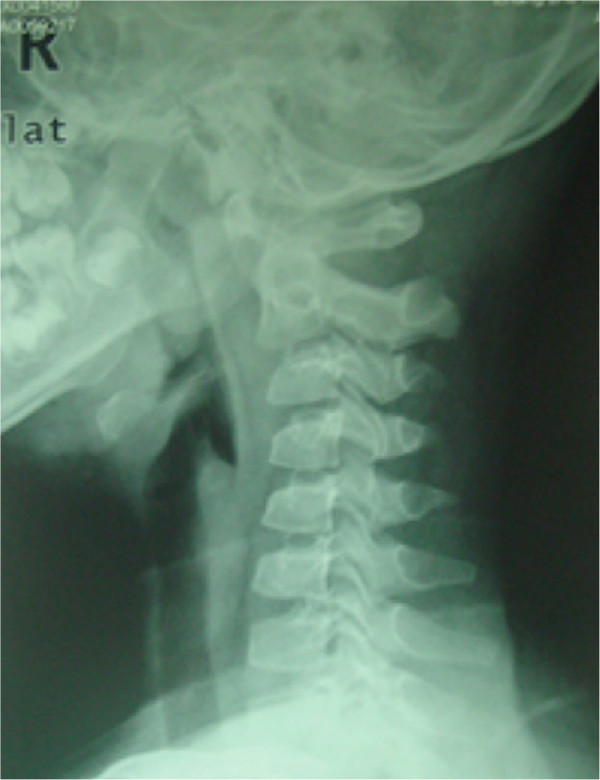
Lateral X-ray showing kyphosis of cervical spine.
